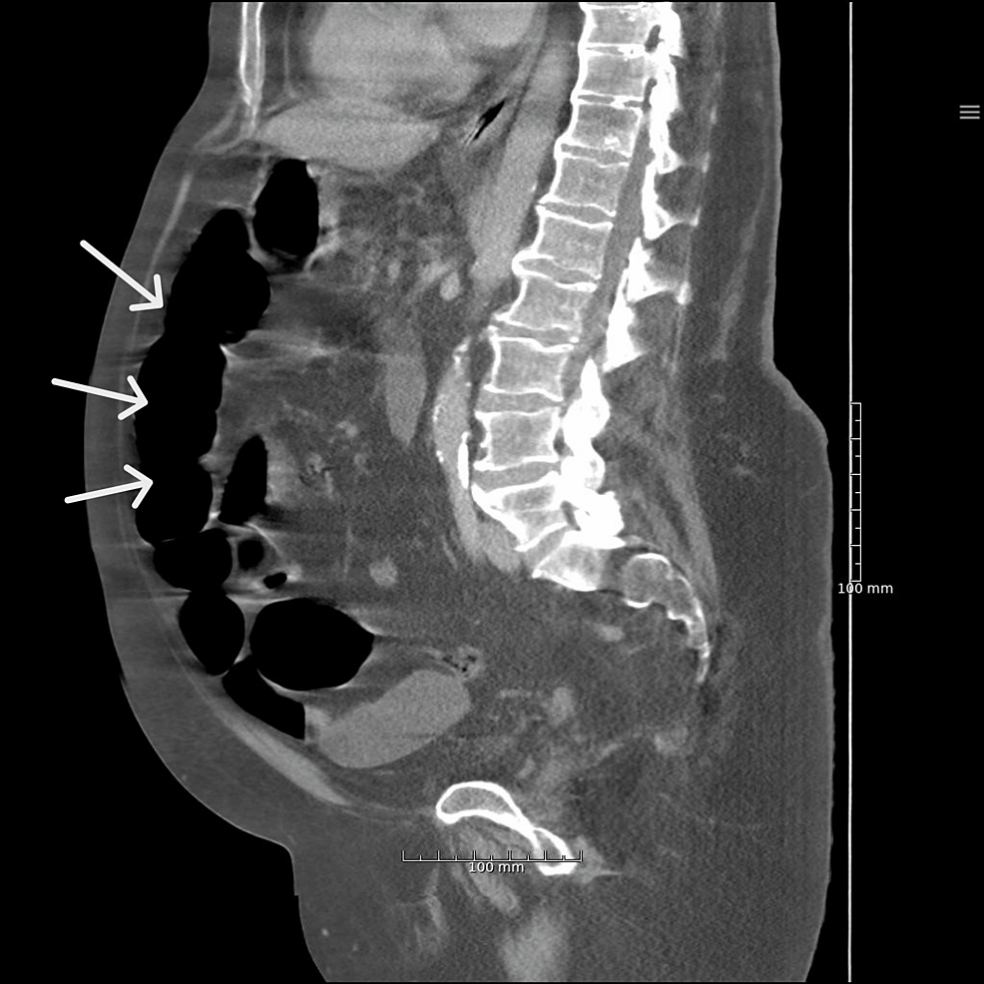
CT abdomen showing small bowel obstruction (SBO) with dilated loops of the small bowel.
